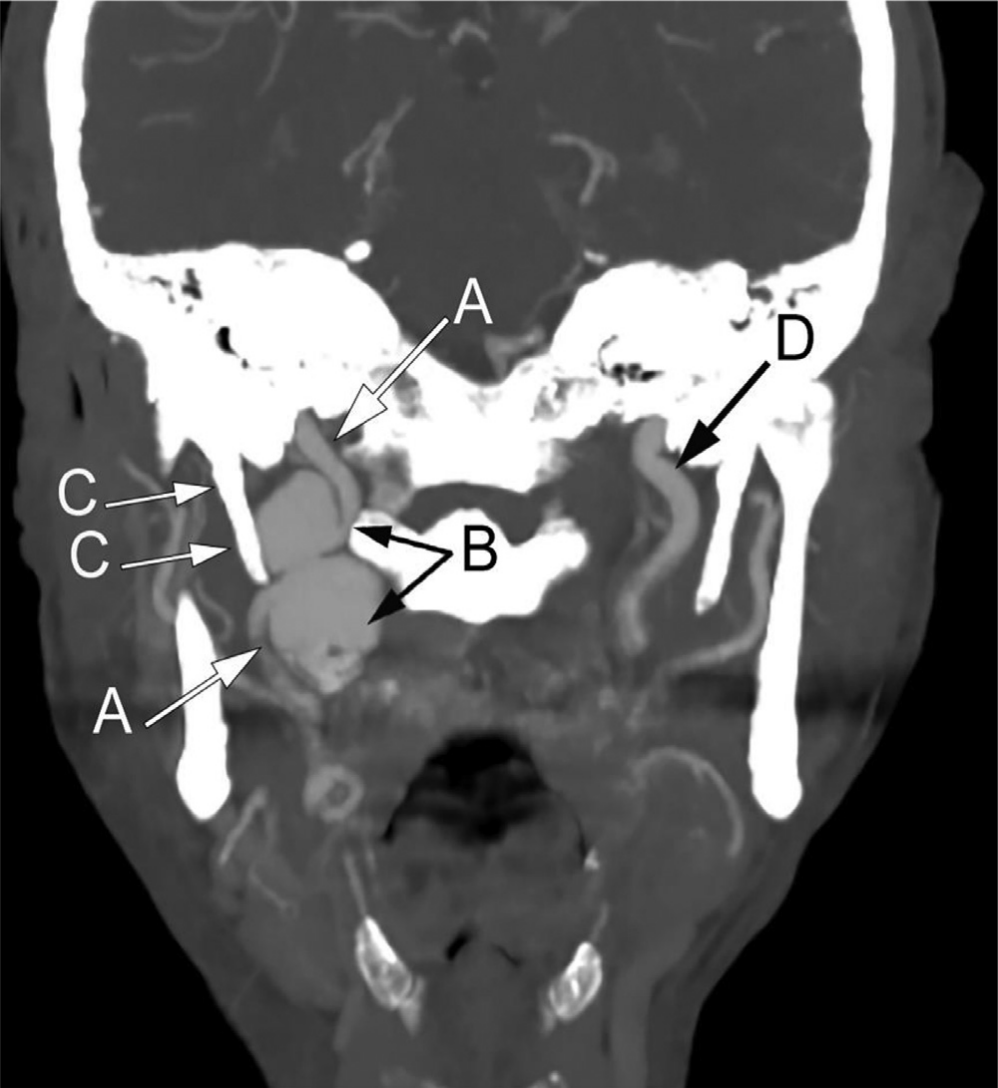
Coronal CT angiographic image demonstrates a normal calibre right internal carotid artery (A) proximal and distal to the aneurysm (B). Note elongated right styloid process (C) which impinges upon the aneurysm waist. Normal left internal carotid artery (D) with prominent left styloid process also present, although this appears remote from the vessel on this side.
